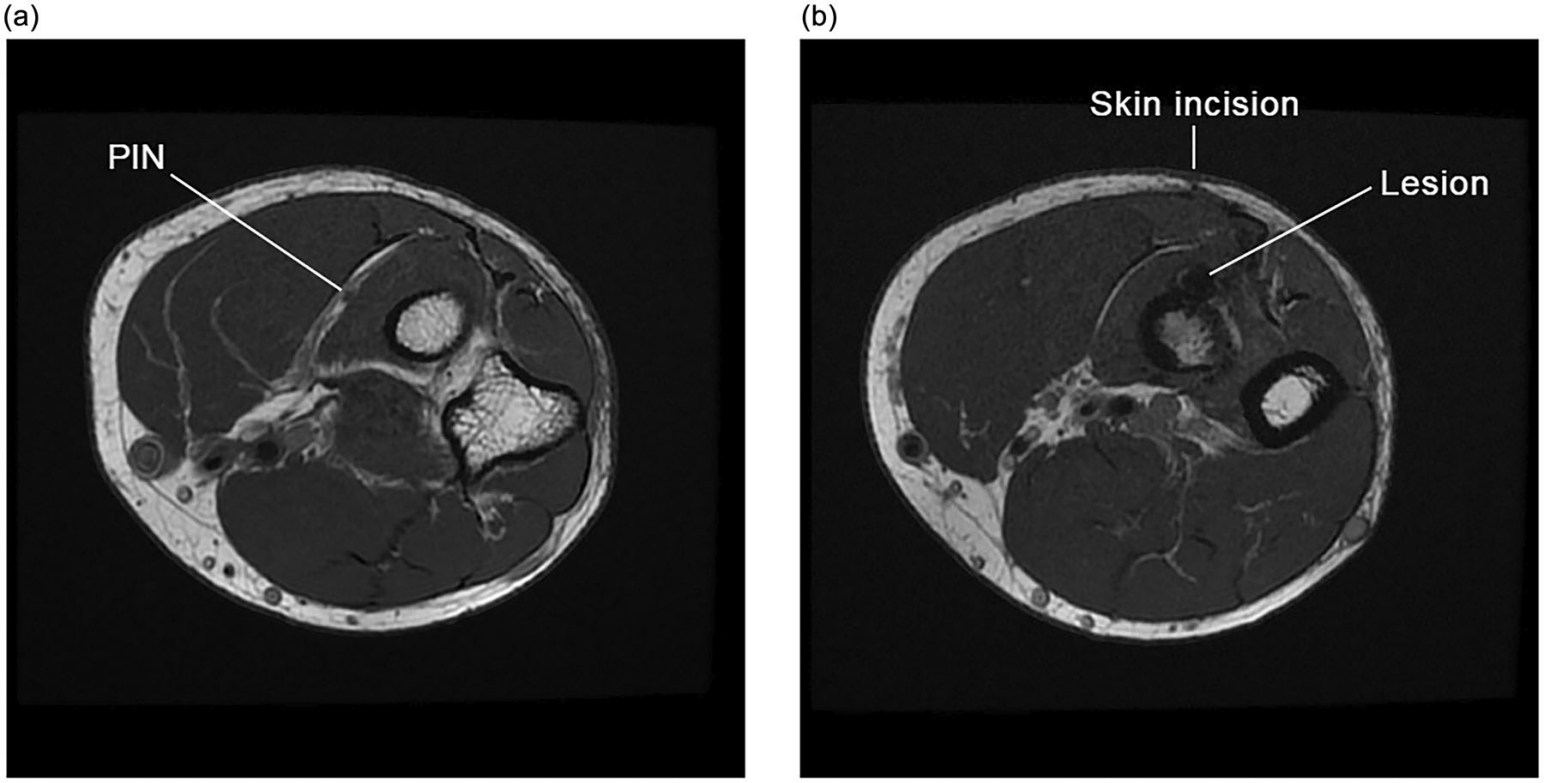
(a,b) Axial T1-weighted magnetic resonance images of the elbow approximately 6 months following a two-incision distal biceps tendon repair demonstrating: (a) an intact more proximal PIN, in its expected location within the fascial plane that will separate the two heads of supinator; and (b) a lesion more distally along the course of the PIN with associated surgical change and suture artifact, along with a more anterior approach than would typically be expected of the posterior incision for a two-incision technique.
